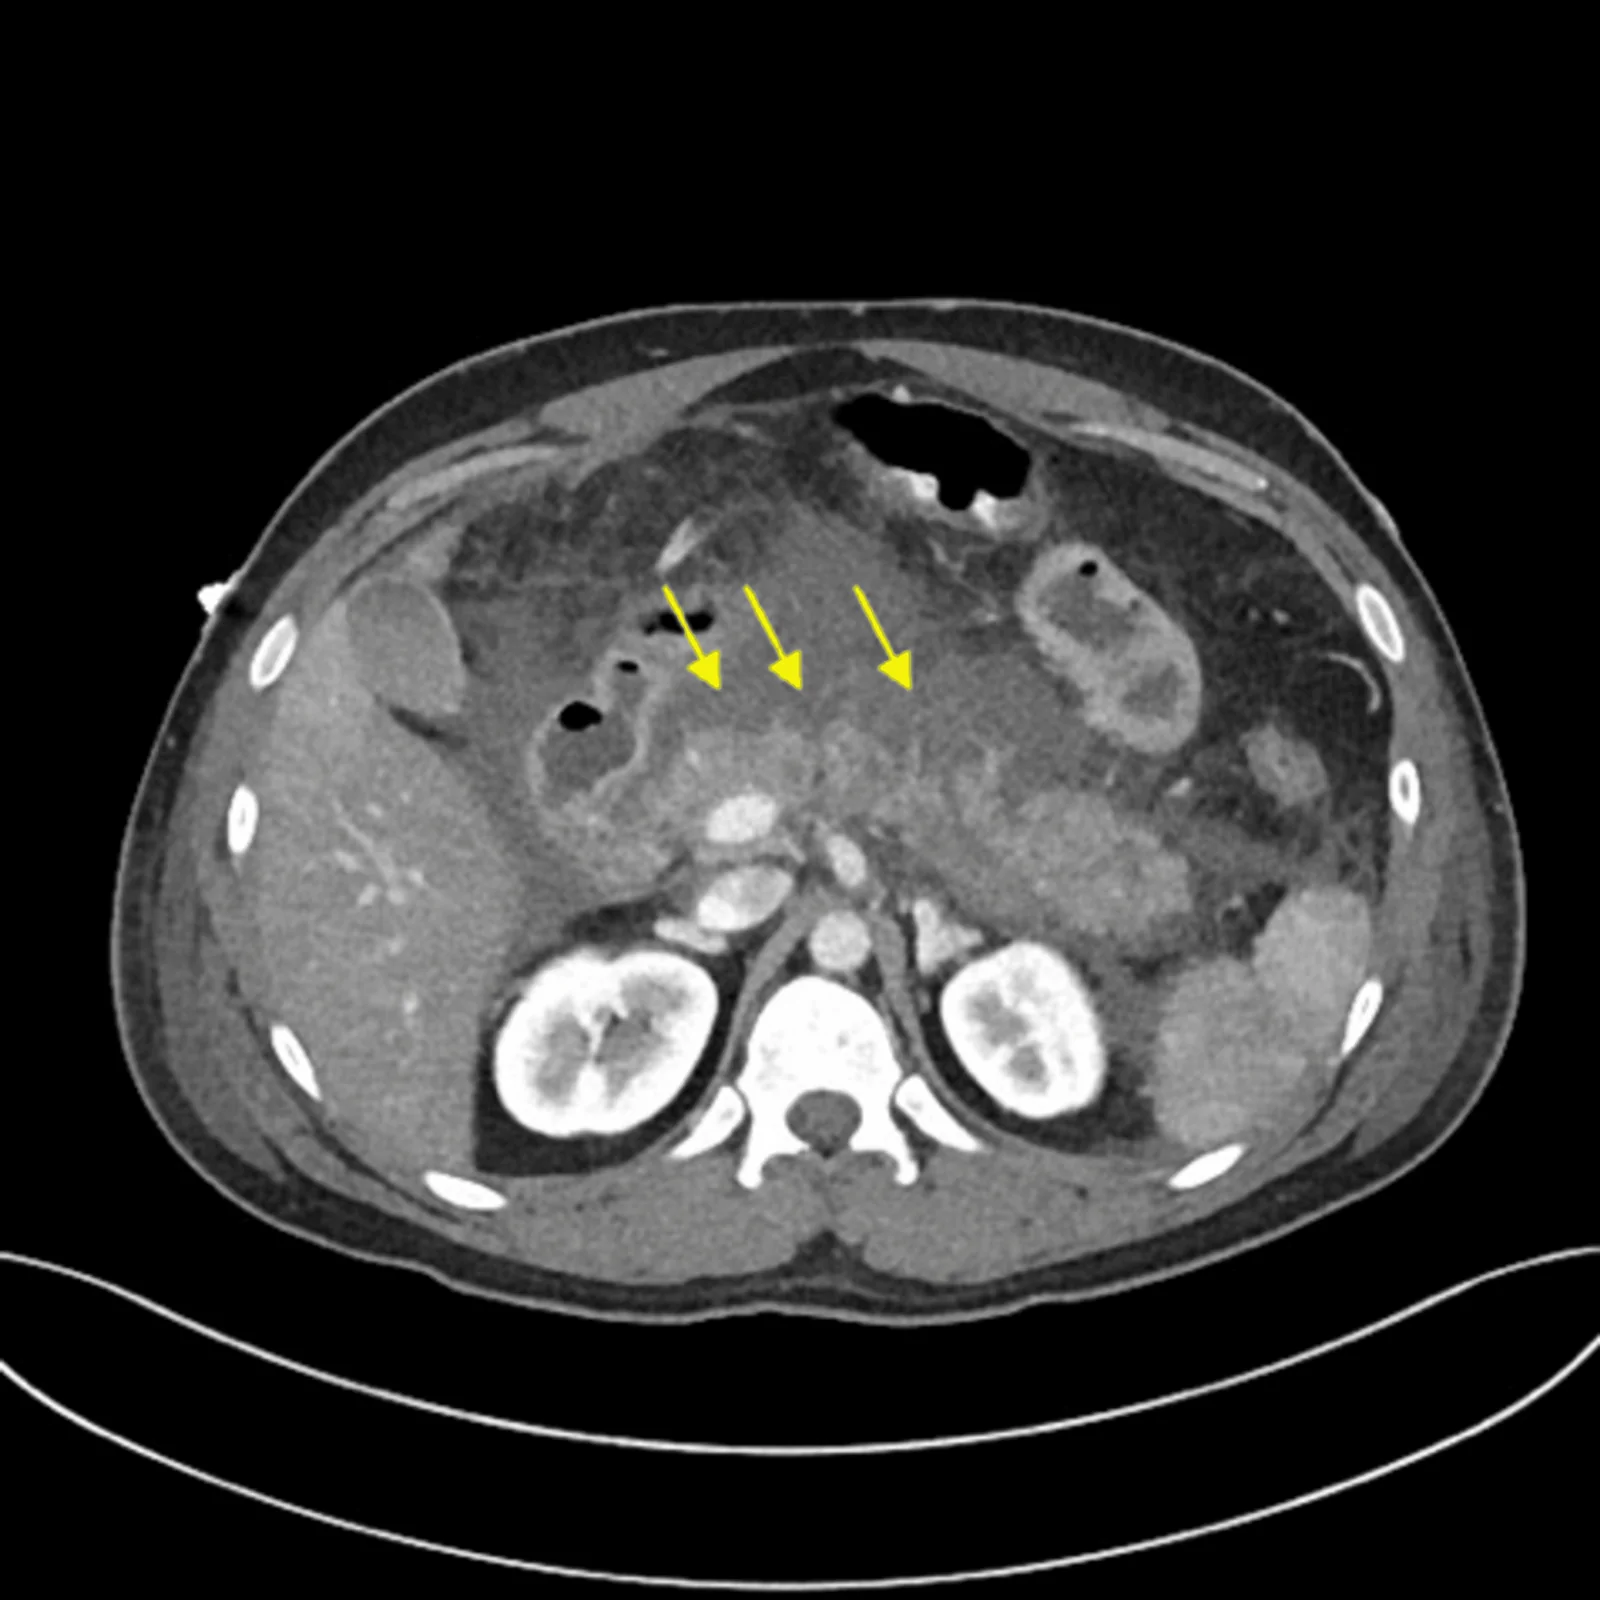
Axial section of a CT scan of the abdomen and pelvis revealing peripancreatic edema (yellow arrows).
